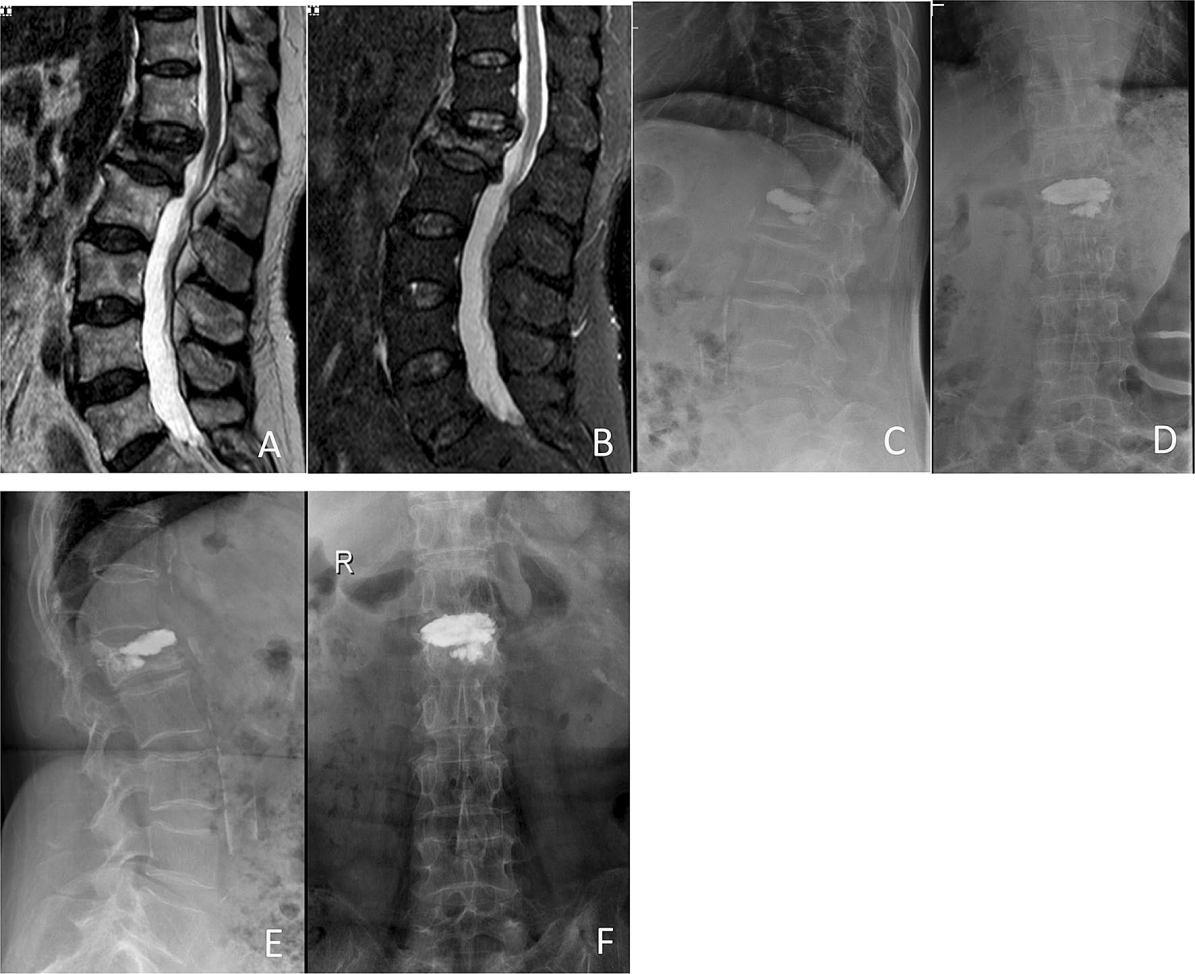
Preoperative and postoperative films of a 60-year-old woman with OVCFs of L1 vertebral body, treated with low-viscosity cement PKP. Preoperative MR image showing the compression fracture at the L1 level (A), (B). Anterior–posterior and lateral film showing the leakage of bone cement into the upper disc space postoperatively (C), (D) and at 1 year postoperatively (E), (F).
